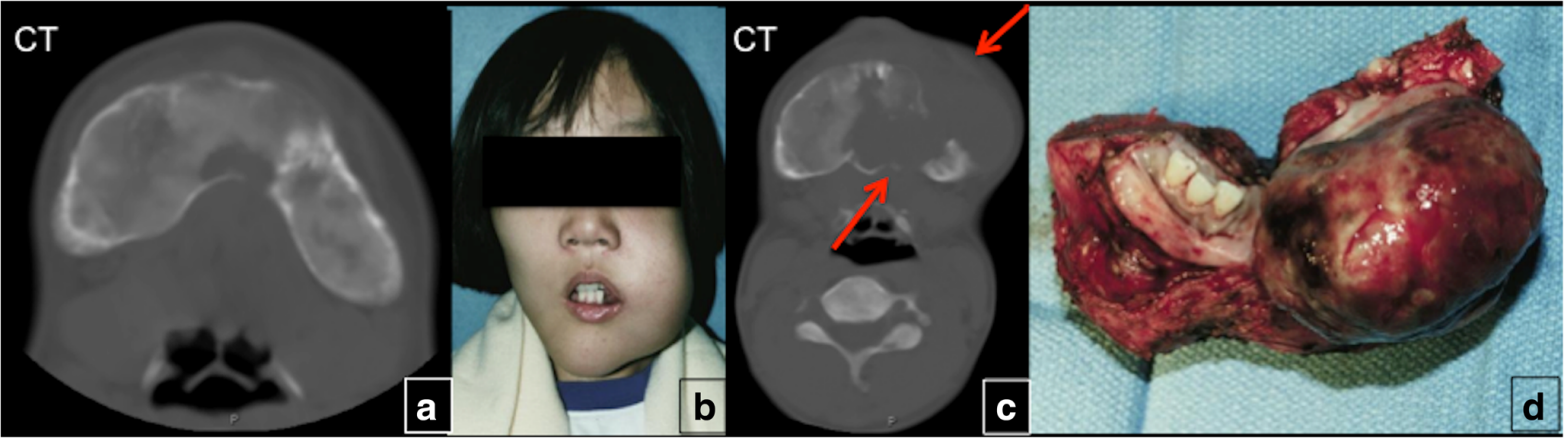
Malignant transformation of the fibrous dysplasia (FD) lesion. A patient with known craniofacial FD (a) presented with enlarging left jaw mass (b). CT of the facial bones demonstrated an aggressive lytic lesion with soft tissue component involving the left aspect of the mandible (*red arrows*) (c). The pathology showed malignant transformation of the mandibular FD lesion
